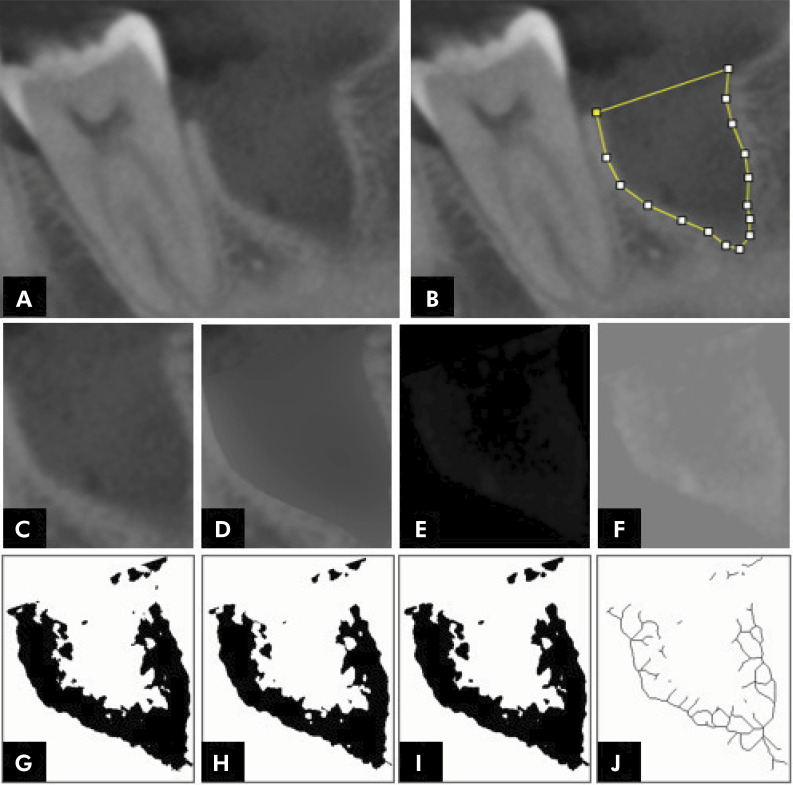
Step-by-step analysis of the fractal dimension of the dental alveoli. A. Coronal slice (5-mm thick) of a CBCT scan; B. Delimitation of the region of interest (ROI) C. ROI (dental socket); D. Blurred image with Gaussian filter; E. Image subtraction result; F. – Addition of a gray value threshold of 128; G. Binary image; H. Eroded image; I. Dilated image; J. Skeletonized image.
